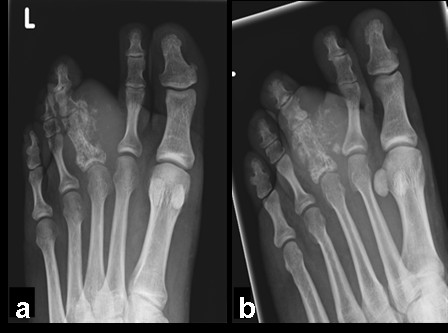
This figure shows anterioposterior (A) and 3/4 (B) conventional radiographs of the left foot. There is a diffuse lesion of the proximal phalanx of the third toe with a moth eaten aspect, cortical destruction and calcifications in the soft tissue.
